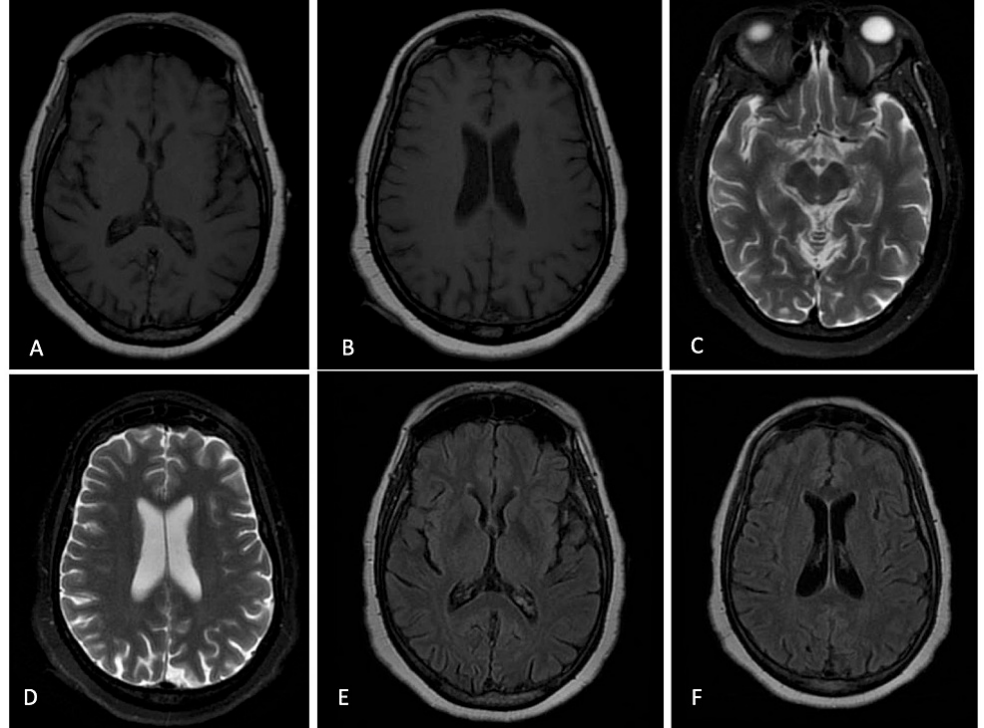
A 60-year-old female with Hashimoto's encephalopathy. Brain MRI was performed at the onset of symptoms. Axial T1 (A and B), axial T2 (C and D), and axial FLAIR (E and F) show no extra-axial fluid collection, intracranial mass, or mass effect. Contrast-enhanced images demonstrate no abnormal intracranial enhancement. All midline structures are completely formed and demonstrate normal morphology. No heterotopic gray matter or cortical abnormality is identified. The ventricular size and brain volume are proportional and age-appropriate. Essentially a negative examination. FLAIR, fluid-attenuated inversion recovery
